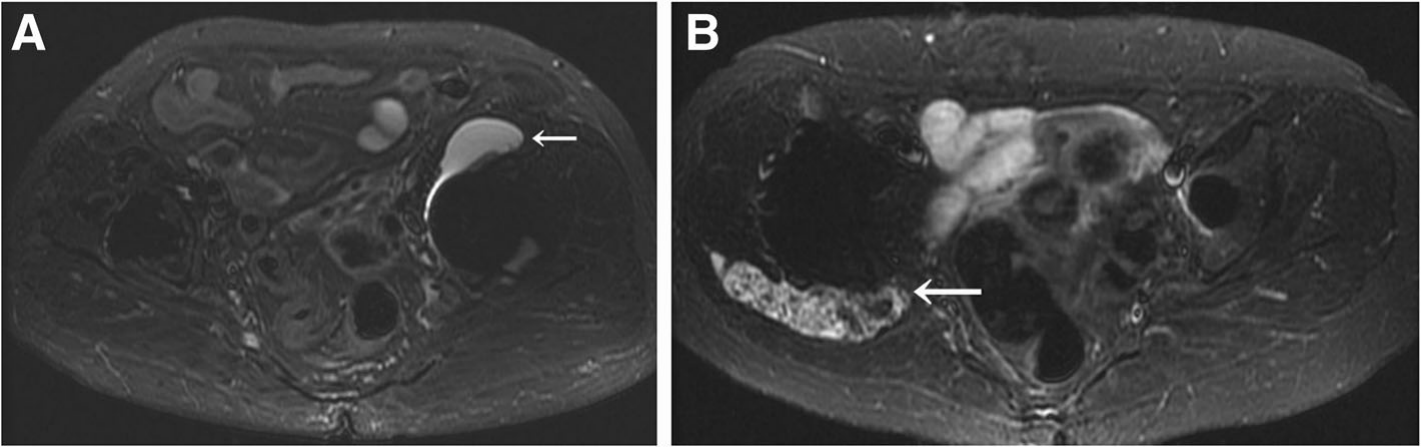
Magnetic resonance images (transverse short tau inversion recovery images) showing pseudotumors (*arrows*) of the cystic type (a) and the mixed solid cystic type (b)
